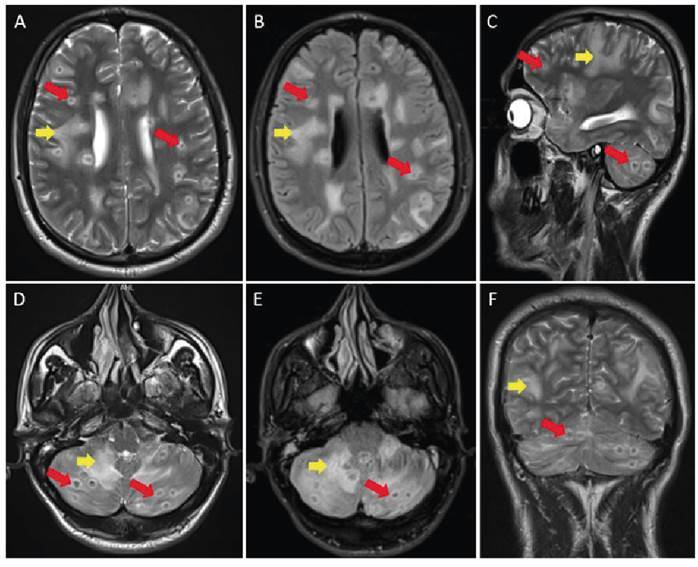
Axial T2-weighted (T2W) (A, D), axial fluid attenuated inversion recovery (FLAIR) (B, E), sagittal (C), and coronal (F) T2W brain magnetic resonance (MR) images showing lesion foci with a centrally hypointense and peripheral hyperintense signal intensity (red arrows), which are numerous at the supra and infratentorial levels, most of which are subcortical, accompanied by peripheral vasogenic edema (yellow arrows) in some regions.
